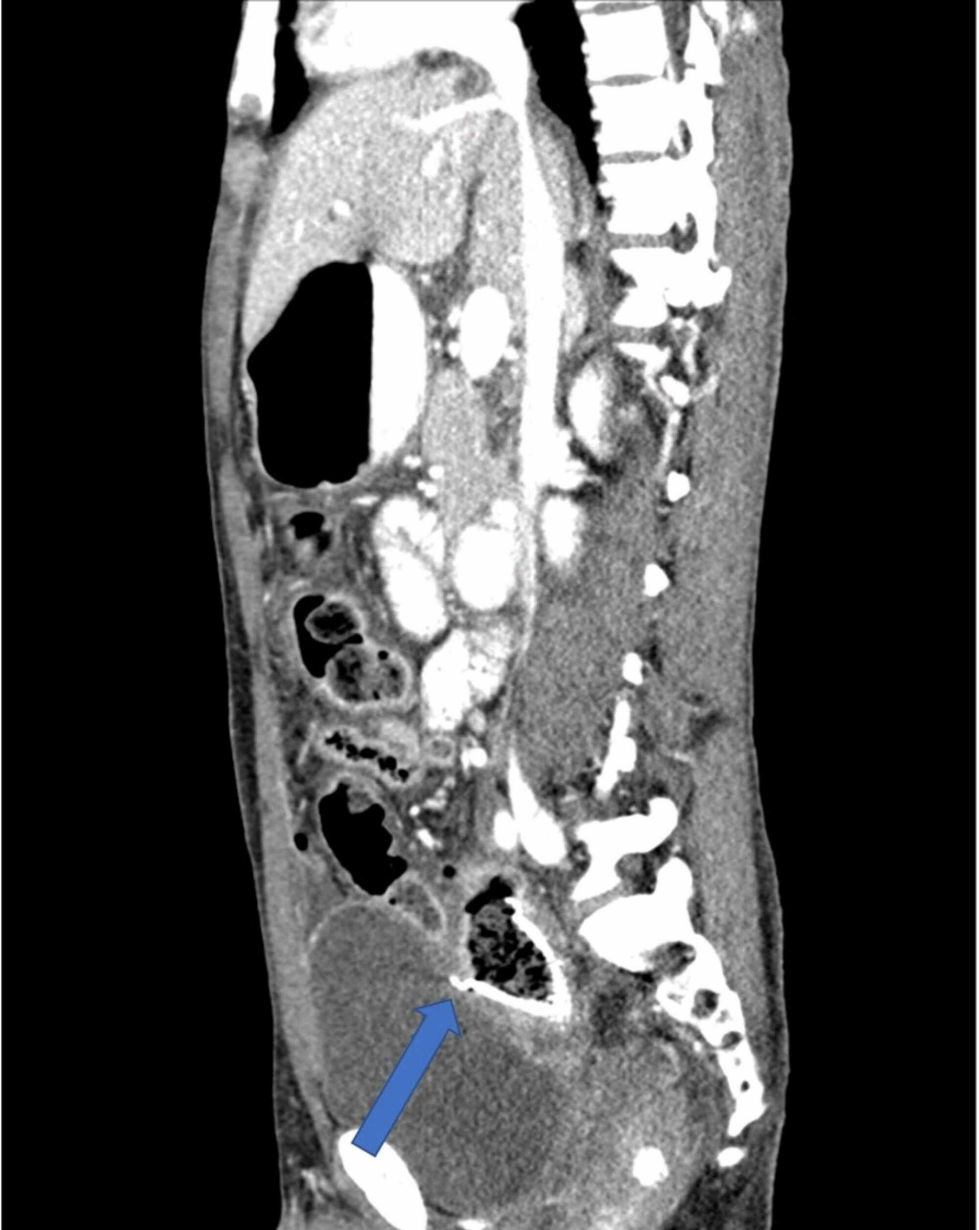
CT scan of the abdomen demonstrating the rectal stent in its place but intending the anterior rectal wall; note the free air in the abdomen (sagittal view)
